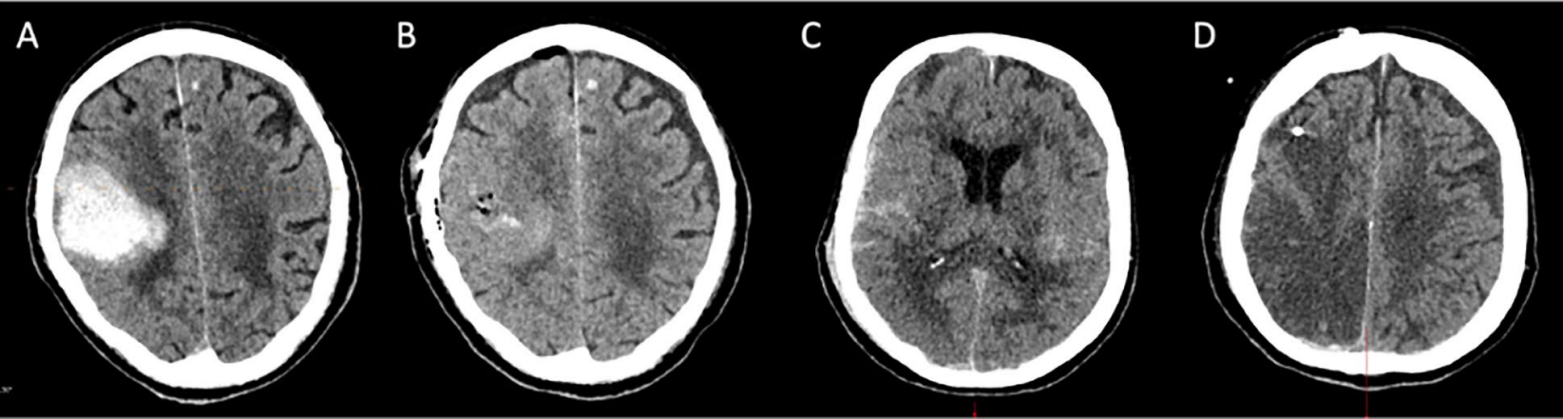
Representative preoperative and postoperative CT scans of two patients. (A) Preoperative CT scan of patient 27, showing a 37 mm parietal intracranial hemorrhage. (B) Postoperative CT scan of the same patient, demonstrating successful hematoma evacuation with no significant residual hemorrhage at the surgical site. (C) Initial CT scan of patient 2 with severe traumatic brain injury. (D) Follow- up CT scan one day after intracranial pressure monitor placement, showing no procedure-related bleeding but revealing an ischemic stroke in the posterior and middle cerebral artery territories. CT, computed tomography
